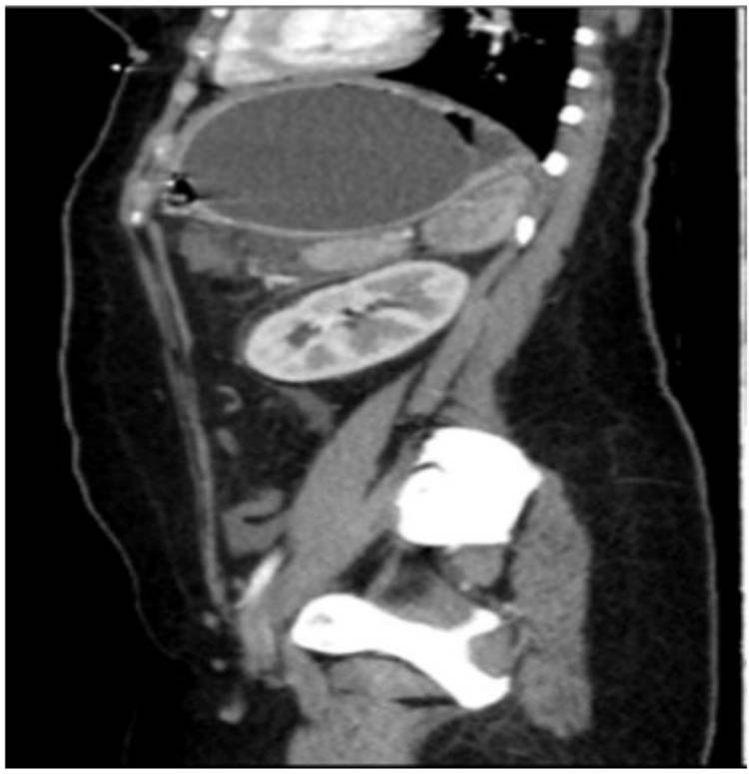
Axial computed tomography (CT) showing IGB compressing the pancreatic body with mild pancreatic edema, regional fat stranding and surrounding fluid collection seen tracking to left perirenal space.
